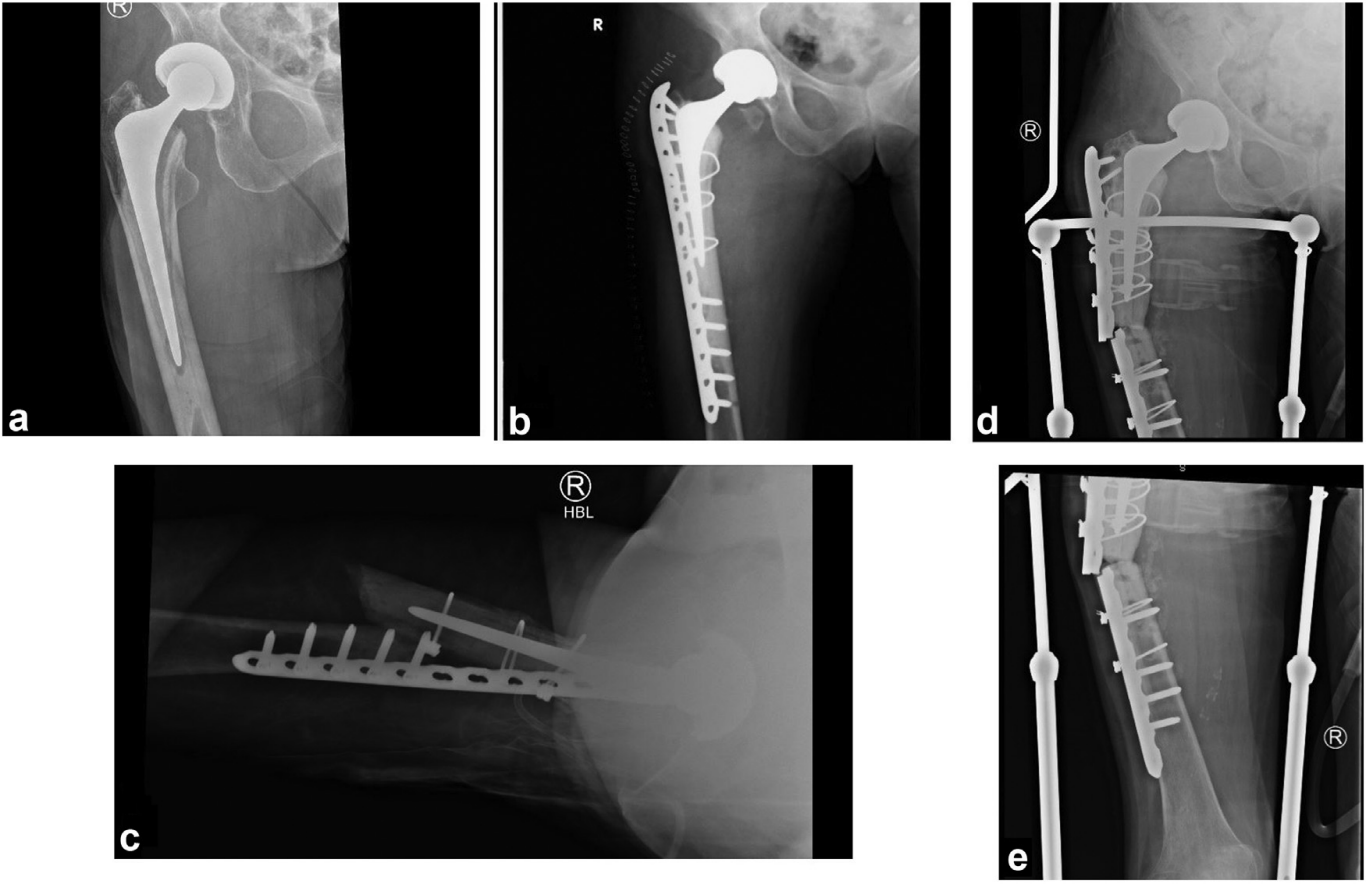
(a) Anteroposterior (AP) radiograph of the periprosthetic fracture in a THA. (b) The same patient after cement-in-cement revision to a short Exeter stem. (c) Cross-table lateral of refracture distal to the short Exeter stem. (d) AP hip of refracture after repeat ORIF and bone grafting. (e) AP distal femur of refracture after repeat ORIF and bone grafting.
